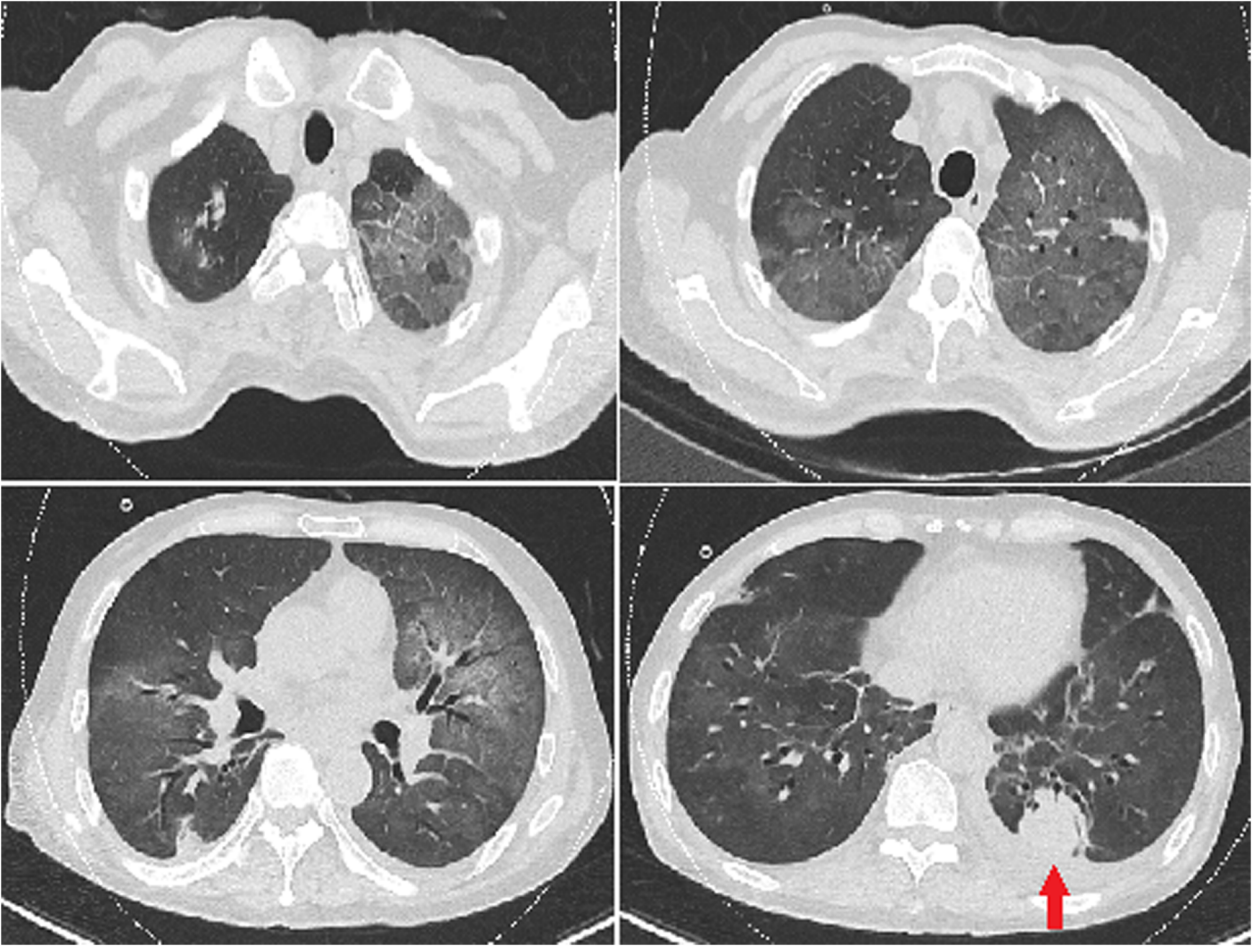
Chest CT showing multiple nodules with diffuse ground-glass opacities were observed in both lungs. First percutaneous needle biopsy of nodule was performed in the left lower lung (red arrow)
